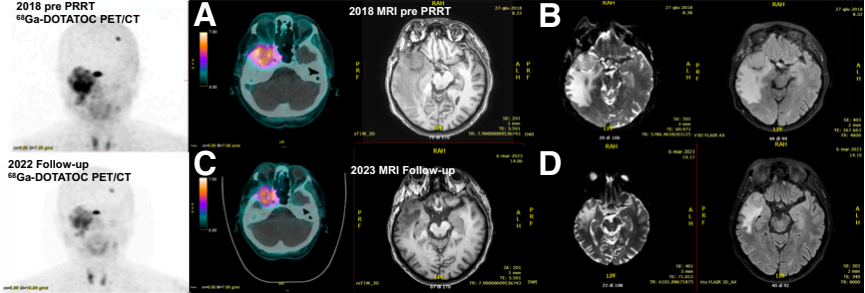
(A) ^68^Ga-DOTATOC PET/CT–positive image of symptomatic patient who underwent 2 resections in 1990 and in 1992. (B) MRI from 2018 of same patient with recurrent and progressive meningioma, who also received radiotherapy (50 Gy/25 fractions) with neither disease nor symptom improvement. After multidisciplinary discussion, decision was made to include patient in our ^177^Lu protocol, and after 5 cycles of ^177^Lu-DOTATATE PRRT, patient obtained partial response with clinical improvement. ^68^Ga-DOTATOC PET/CT performed in 2022 (C) showed persistent positivity and partial response that was confirmed at last MRI on June 3, 2023 (D).
